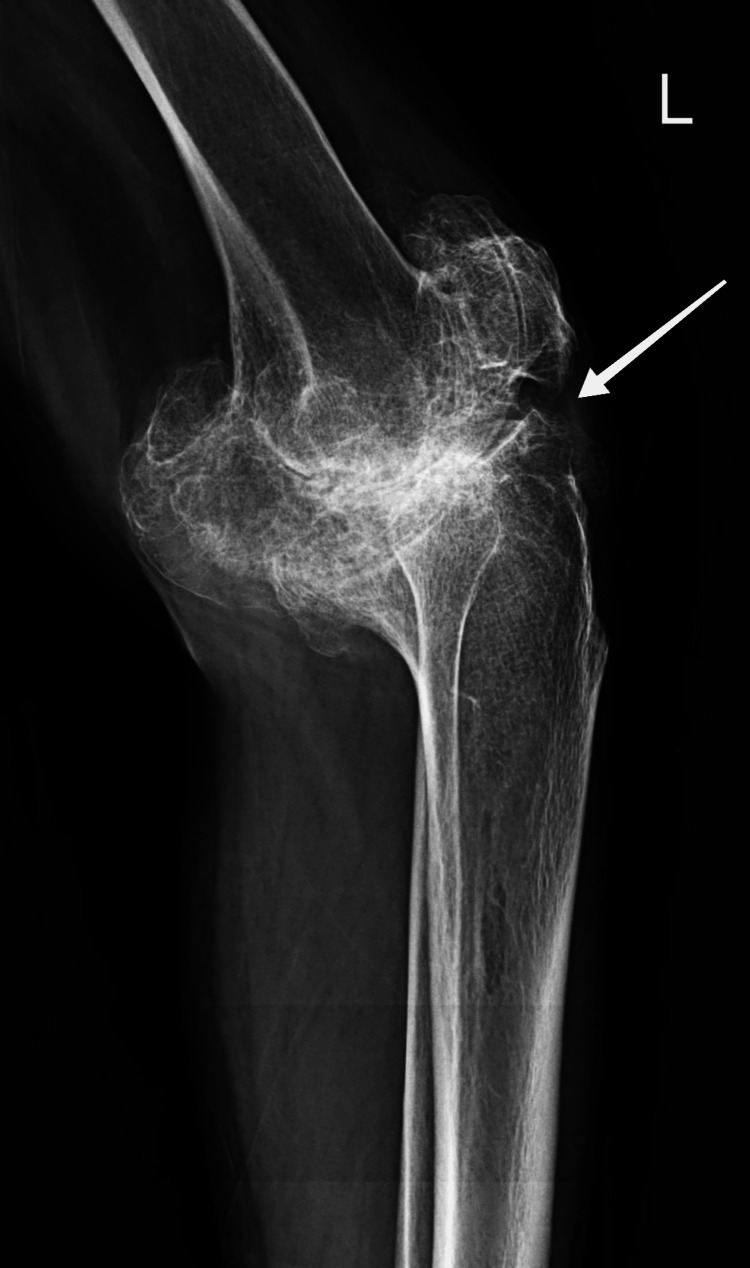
Pre-operative left knee X-ray.
